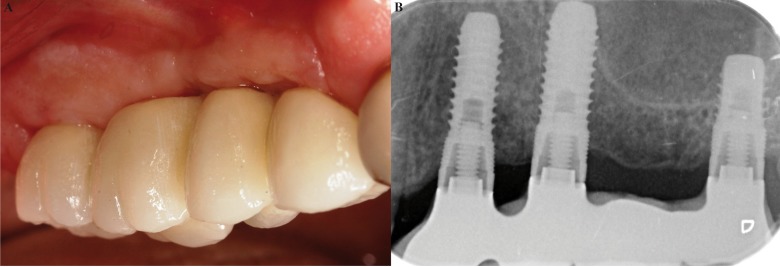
A) Placement of the finished prosthesis; B) Radiographic check-up after 12 months.
